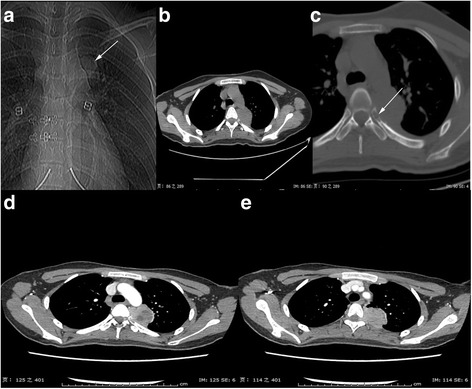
a X-ray revealed a mass located in the left mediastinal. Non-contrast b, c and contrast-enhanced d, e chest CT showed a heterogeneous mass deeply situating in the posterior mediastinum and enhancing inhomogeneously. The mass showed an obvious extension through the intervertebral foramen. d Compression of the lung and invasion of the adjacent rib are visualized
